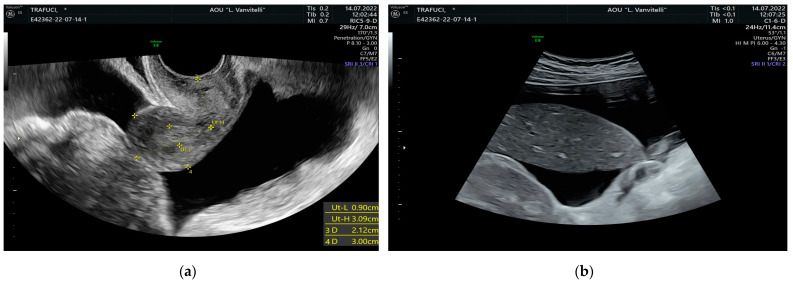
Transvaginal ultrasound image of massive ascites: (a) in the pouch of Douglas; (b) in the perihepatic space.
